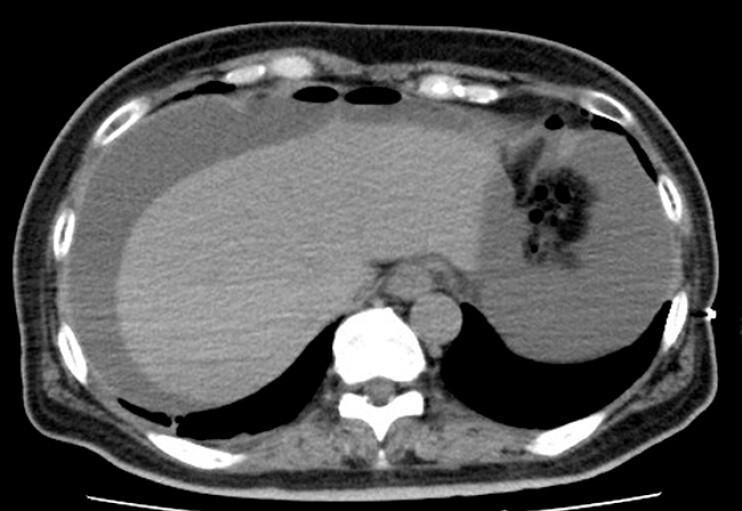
Abdominal CT image when visiting our institution. Abdominal CT showed free air and ascites on the liver surface. Abbreviation: CT, computed tomography.
